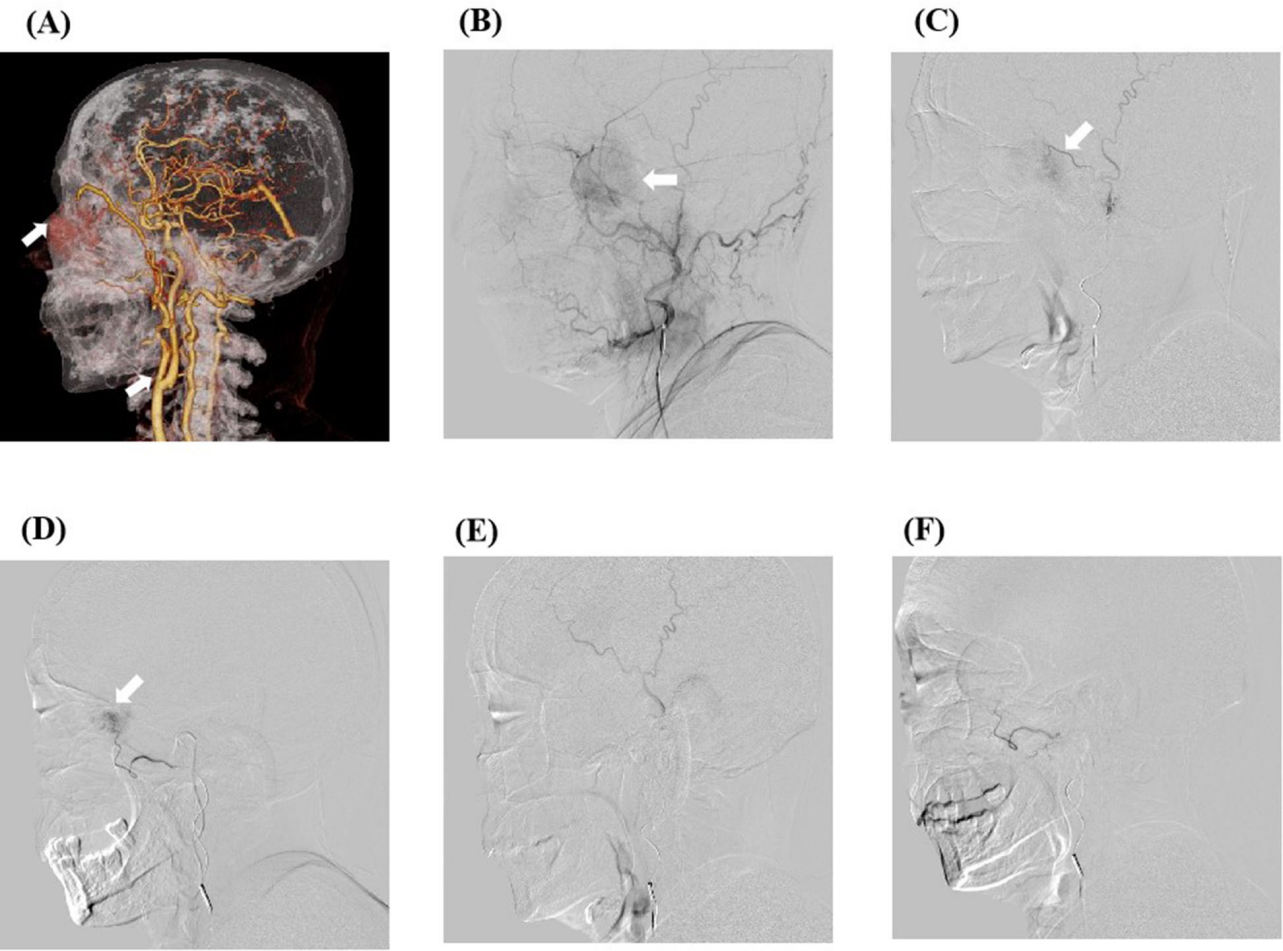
The preoperative arteriography results of the patient and the digital subtraction angiography (DSA) images of transcarotid chemoembolization. (A) The preoperative computed tomography angiography results of the patient show that the mass was supplied by the external carotid artery branch. (B) DSA image of the patient’s frontotemporal mass before embolization. After anesthesia, imaging examination through the left distal radial artery approach revealed an abnormal vessel with a diameter of about 3.5 cm on the left side of the face. A microcatheter was inserted into the external carotid artery branch, and 0.3 mg of gemcitabine was administered. The microspheres were then embolized with polyvinyl alcohol (blue spheres 100–300 microns in diameter) containing 0.5 g (1000 mg/m^2^) gemcitabine. (C, D) Intraoperative superselective arterial DSA images. (E, F) DSA images of the patient after embolization show that the tumor is not visible.
